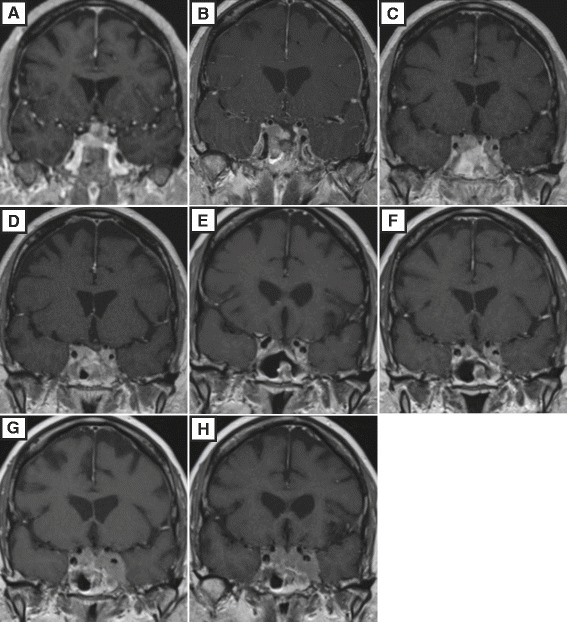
MRI of the pituitary throughout the patient’s clinical course. Coronal T1-weighted post-gadolinium images are shown. After partial resection at another facility, she presented to us for more definitive endoscopic endonasal resection of the residual sellar tumor (a), which was successful (b). She then underwent radiation therapy 3 months after this repeat surgery, at which point her cavernous sinus residual disease had already subtly progressed (c). Radiation therapy was initially successful for local control of disease 3 months after completion of fractionated IMRT (d). Two months following this MRI, her ACTH levels increased dramatically despite stability of her cavernous sinus residual disease (e), and metastasis to the liver was diagnosed (see Fig. 4). Six months after resection of the liver metastasis, her sellar and cavernous sinus disease remained stable (f), but then quickly progressed over the next 6 months (g). She was started on dual-agent oral chemotherapy, and 8 months later her local disease was stable (h), although she has suffered additional liver metastases
